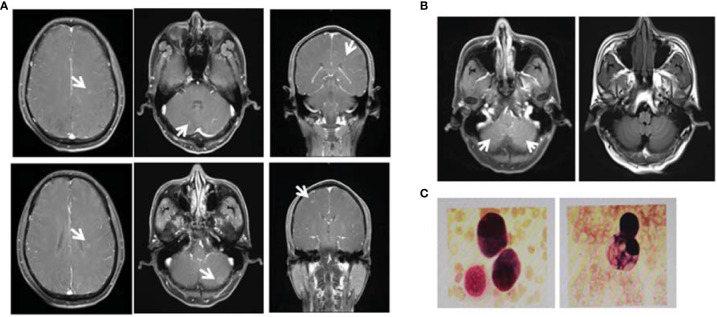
Diagnosis of brain metastases. (A) On October 31, 2019, the magnetic resonance imaging (MRI) examination of the patient showed multiple brain masses including multiple metastases in the lateral ventricle, right frontal lobe, and cerebellar hemisphere. Diagnosis of leptomeningeal metastases. (B) On April 21, 2020, MRI T1 enhancement and FLARE examination showed that the cerebellar sulci had multiple line-like and spot-like enhancements, suggesting meningeal metastasis. (C) On April 30, 2020, retest of the cerebrospinal fluid (CSF) showed the presence of cancer cells with the characteristics of adenocarcinoma cells.
